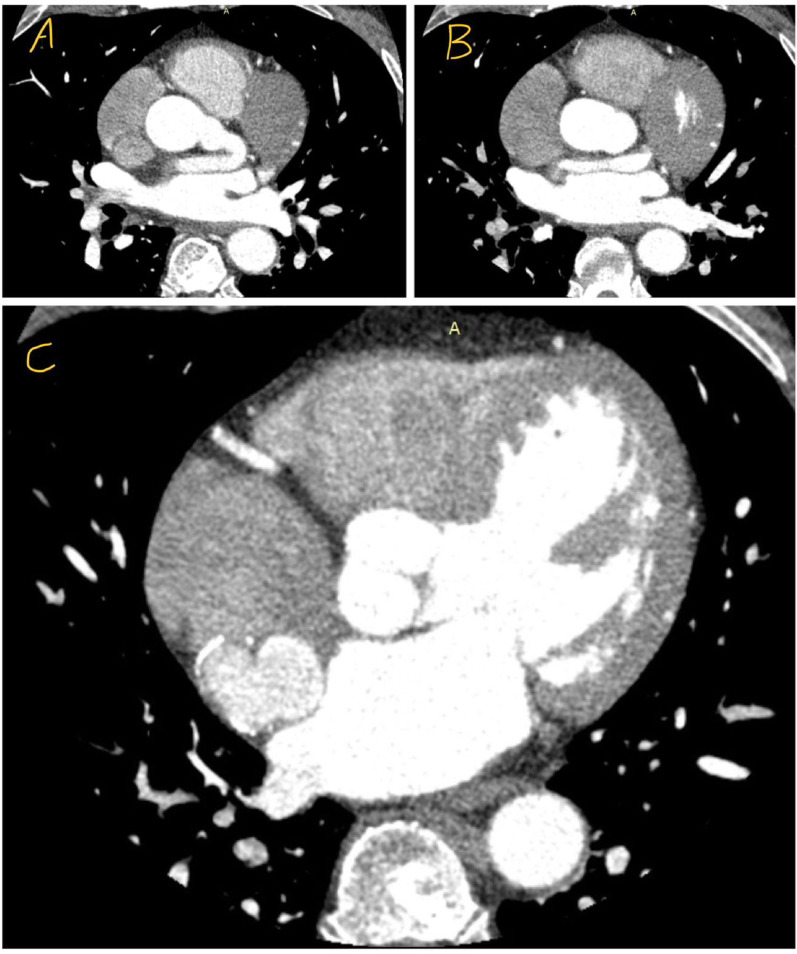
CT coronary angiography demonstrating a giant left main coronary artery aneurysm with fistulous drainage to the right atrium
